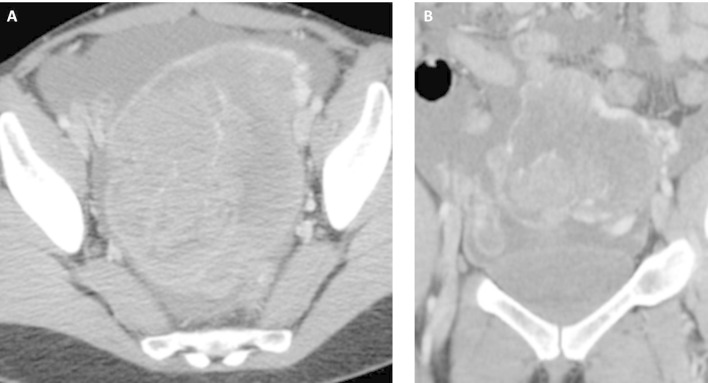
Axial (A) and coronal (B) scans of abdominal CT which shows ovarian tumor at
